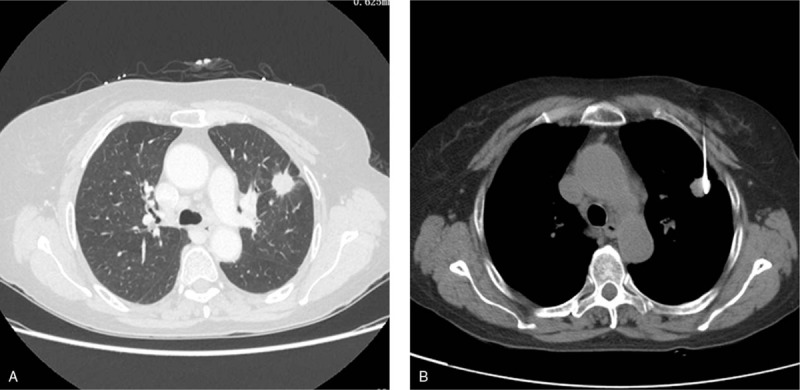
A 70-year-old female underwent CT-guided PCNB for small lung nodule (adenocarcinoma). A, CT scan demonstrated the small lung nodule at left upper lobe. B, The lung nodule was punctured for biopsy. CT = computed tomography, PCNB = percutaneous cutting needle biopsy.
